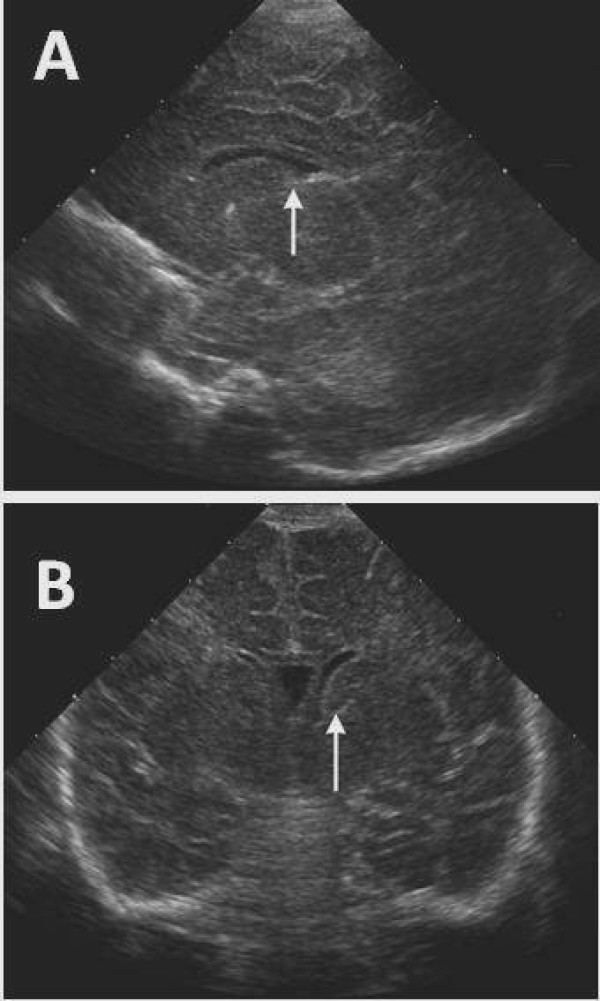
Ultrasound images of the head of a 2 week old normal term infant scanned with an 8V5 ultrasound probe on an Acuson Sequoia 512 ultrasound machine (Siemens, Erlanger, Germany). A: Sagittal view, arrow indicates the caudo-thalamic groove used as a reference point. B: Coronal view, again arrow referencing the normal caudo-thalamic groove.
